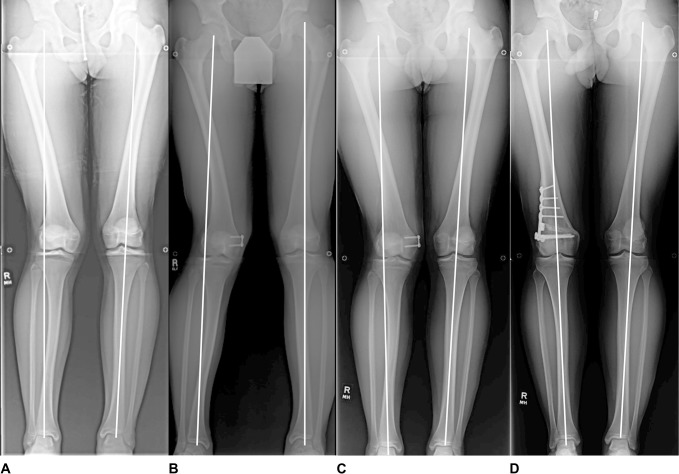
Serial standing alignment radiographs of an adolescent boy (patient #2) with lateral distal femoral hemiphyseal arrest treated unsuccessfully with guided growth and requiring a distal femoral osteotomy to realign his mechanical axis. A, demonstrates the patient’s presenting radiographs. B, represents the patient’s radiographs 6 months after starting guided growth with a medial tension band. C, represents the patient 9 months after initiation of guided growth with no improvement in his mechanical axis. D, represents the patient 6 months after undergoing a lateral opening wedge osteotomy with resolution in his mechanical axis.
